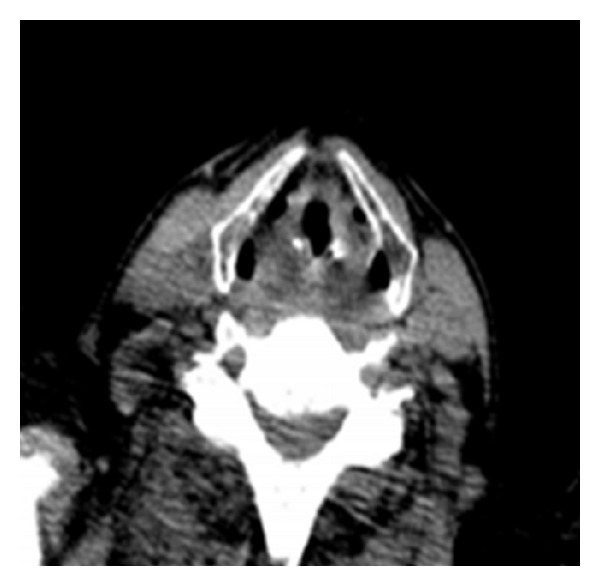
Computed tomography scan of the neck. The images obtained showed increased size of the soft tissue on the lingual surface of the epiglottis.
